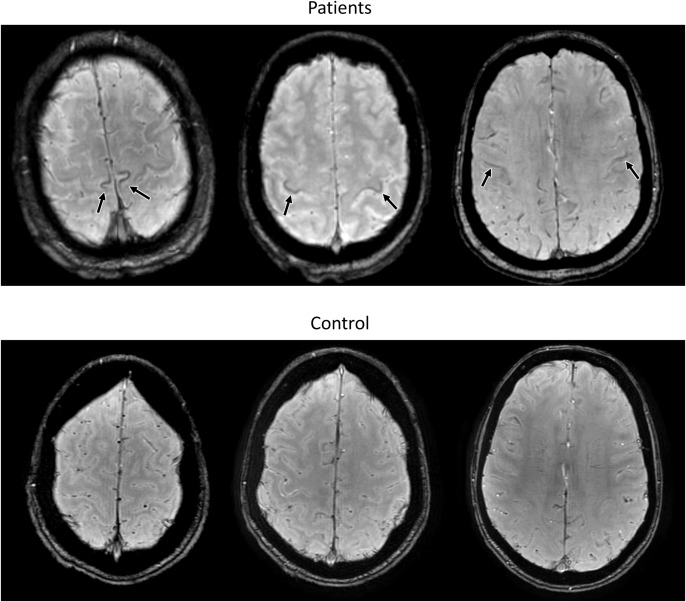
Susceptibility Weighted MR images from 3 different exemplary ELA patients (upper row) and a control subject (lower row) for comparison, evaluated in our center. Note the iron-related hypointensities in the motor cortex at the lower limbs, upper limbs, and bulbar region (from left to right) in the patients with ELA.
